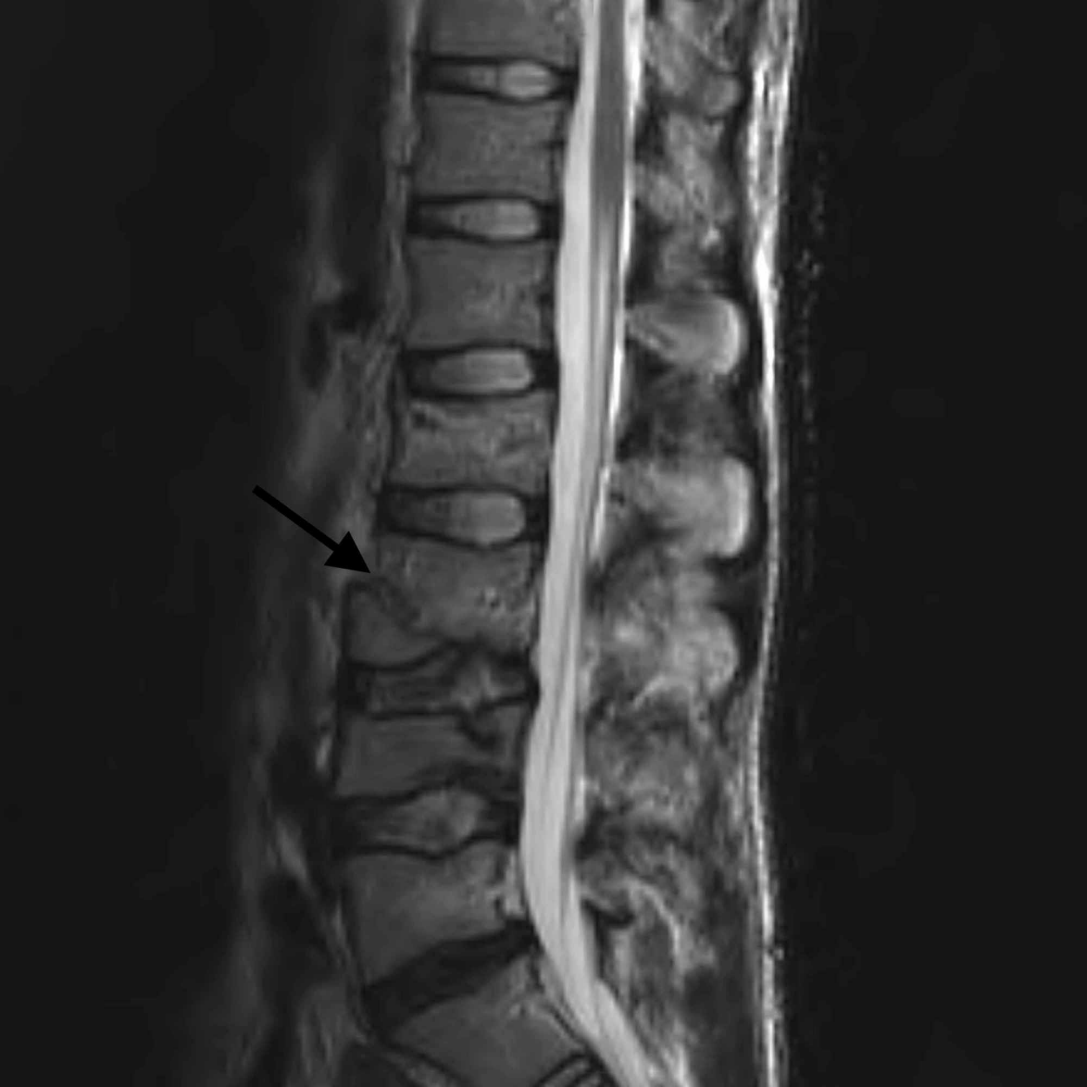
Sagittal T2 MRI showing disruption of the anterior longitudinal ligament (ALL).
